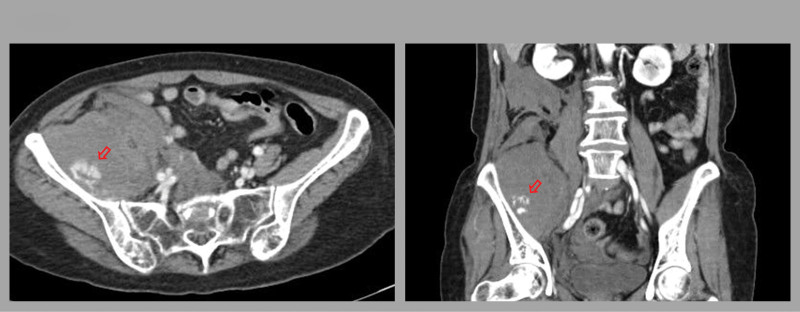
Contrast-enhanced abdominal computed tomography image of iliacus muscle hematoma. Fluid collection with contrast leakage (red arrow) was observed inside the right iliacus muscle (left: transverse view, right: coronal view).
